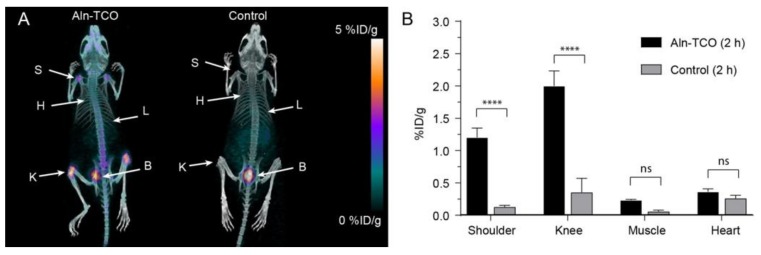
Pretargeted PET/CT bone imaging of [^68^Ga]3 targeting TCO functionalized alendronate (4), which was administered 1 h prior to the administration of [^68^Ga]3. (A) Respective PET/CT images after 2 h. (B) Image-derived quantitative analysis of tissue uptake.  *p* < 0.0001.
